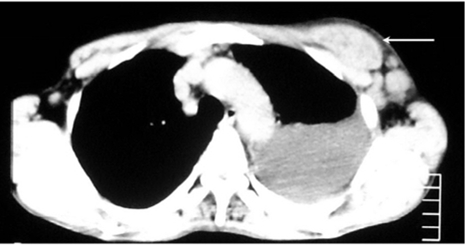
CT scan through the thorax, showing the chest wall mass (arrow).
